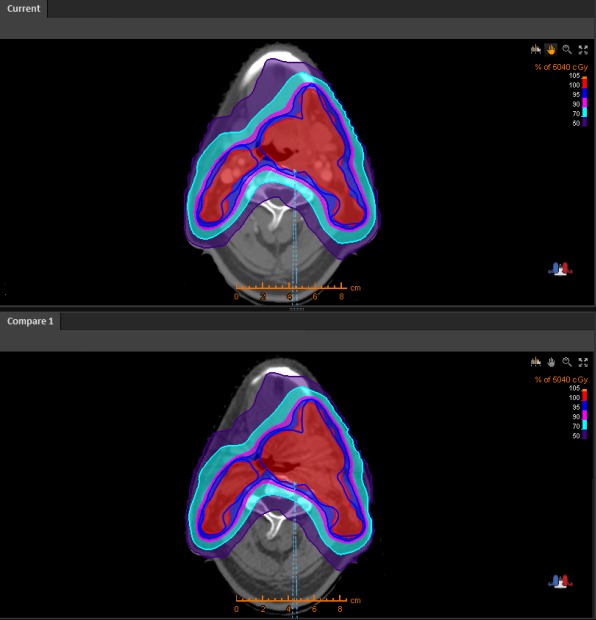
Planning CT (top) and CBCT (bottom) for a patient with head and neck cancer. Blue lines indicate PTV, and the color wash indicates the percentage of the prescription dose.
